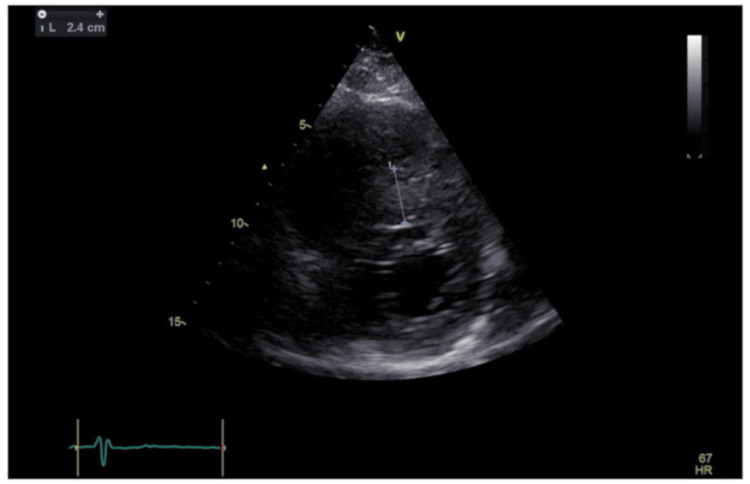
Echocardiogram- parasternal short-axis at the apical level Marked asymmetric septal hypertrophy measuring 2.4 centimetres.
